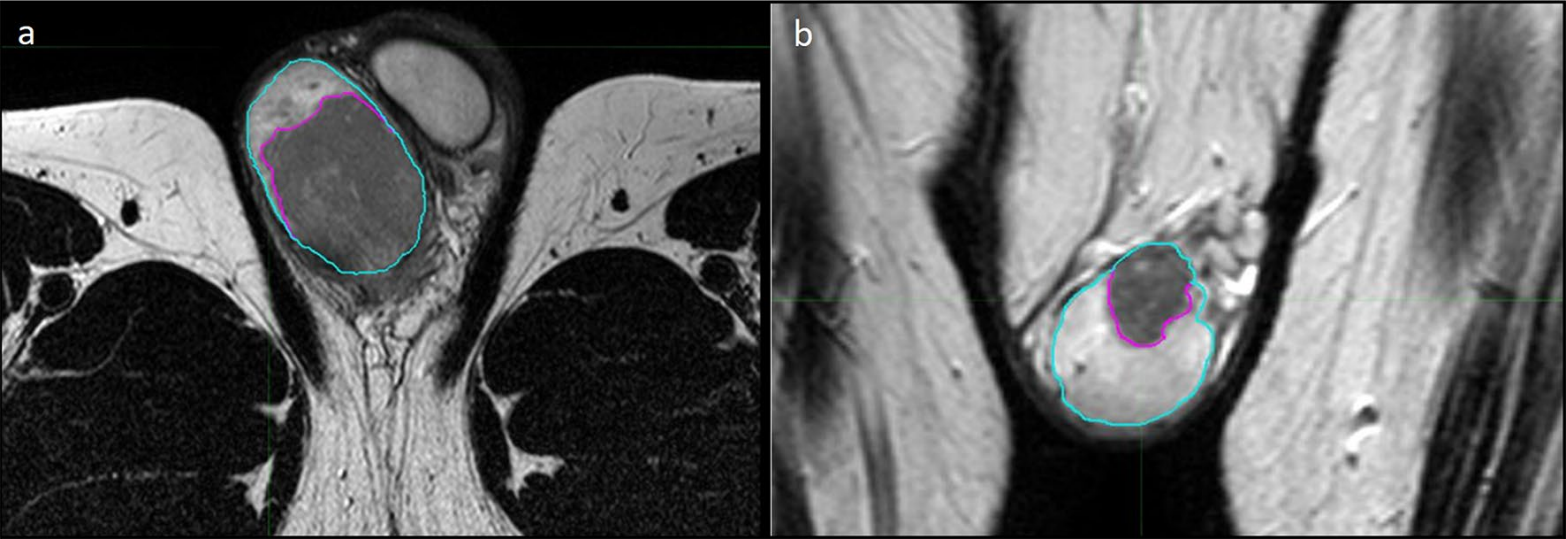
MR images showing the segmentation process in a 27-year-old man with testicular seminoma (a) and a 31-year-old man with Leydig cell tumor (b), axial and coronal T2-weighted images, respectively. Testicles are contoured in blue, whereas neoplasms are contoured in violet.
